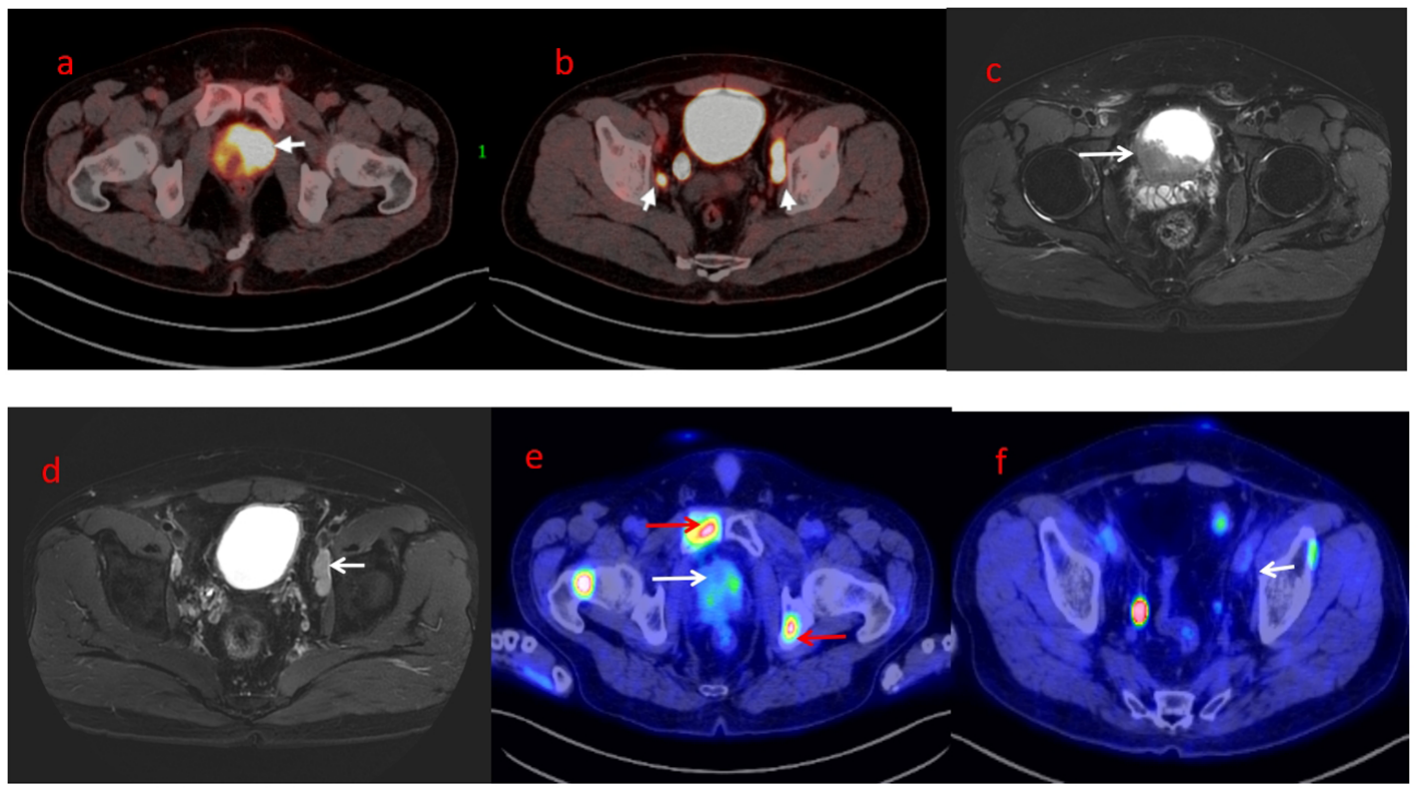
The mass infiltrates the whole prostate and the right lateral wall of the urinary bladder. The right lower ureter was infiltrated by the lesion. Large lymph nodal mass was also seen in the pelvis with multiple retroperitoneal lymphadenopathies (a&b, white arrow). Pelvic MRI showed suspicion of prostate cancer, invasion of the bladder base and seminal vesicles on both sides, multiple enlarged lymph nodes in the bilateral pelvis, and retroperitoneal lymph node metastasis (c&d, white arrow). The PET-CT 8 months after PBT showed that the prostate lesions were basically under control and the metastatic lymph nodes in the pelvis were significantly reduced (e&f, white arrow). PET-CT showed multiple bone metastases (e, red arrow).
